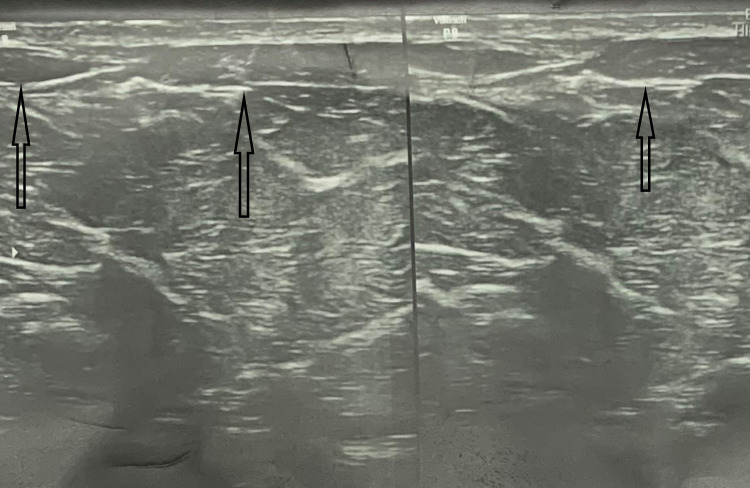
Ultrasound showing dilated superficial vein of the chest wall and breast
